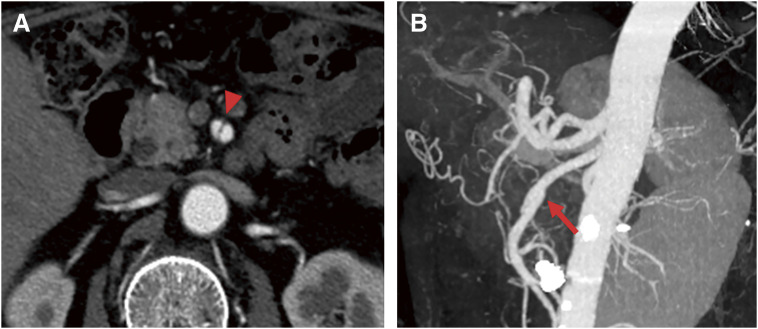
Follow-up computed tomography 2 years after endovascular treatment. (A) Axial view at the pancreatic level: The dissection of the superior mesenteric artery persists (arrowhead). (B) 3D reconstruction of the abdominal vasculature: The false lumen remained partially patent (arrow) but had shrunk significantly compared with Fig. 1B, and no additional intervention was required.
